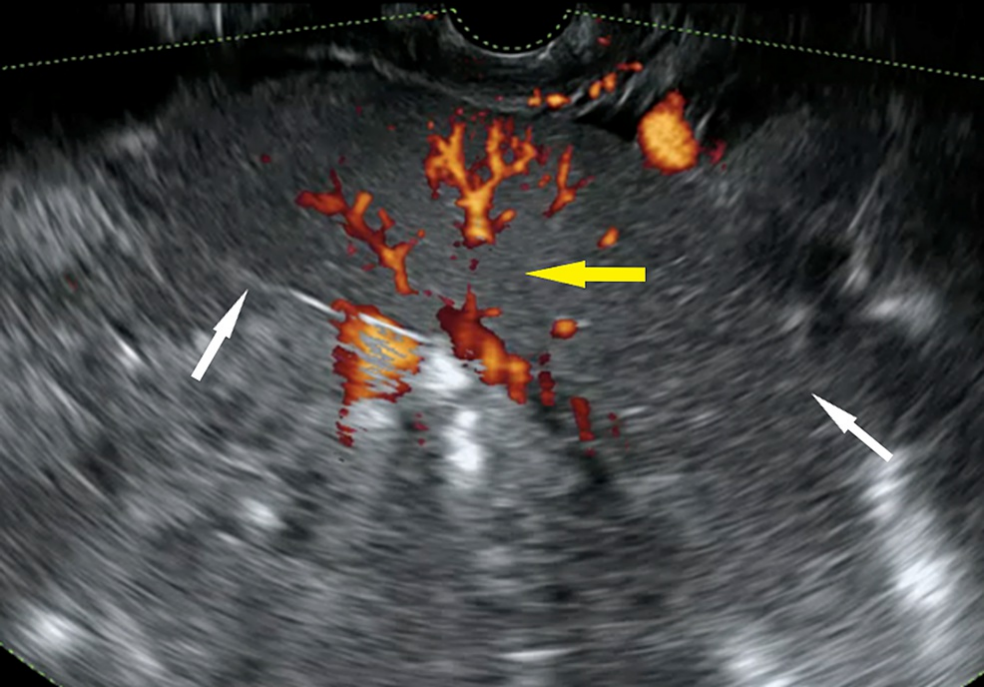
Doppler-enhanced transvaginal ultrasonography revealed central vascularity (yellow arrow) of the lesion (white arrows)
